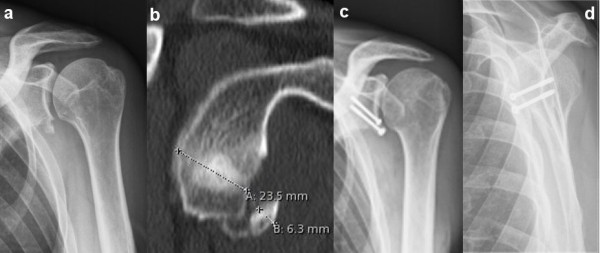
Case example of a large glenoid rim fracture and screw fixation. 69 year old female with traumatic anterior shoulder dislocation. (a) The conventional x-ray examination showed an osseous bankart lesion with an estimated defect size of 21% (b). Open reduction and internal screw fixation has been performed (c, d).
